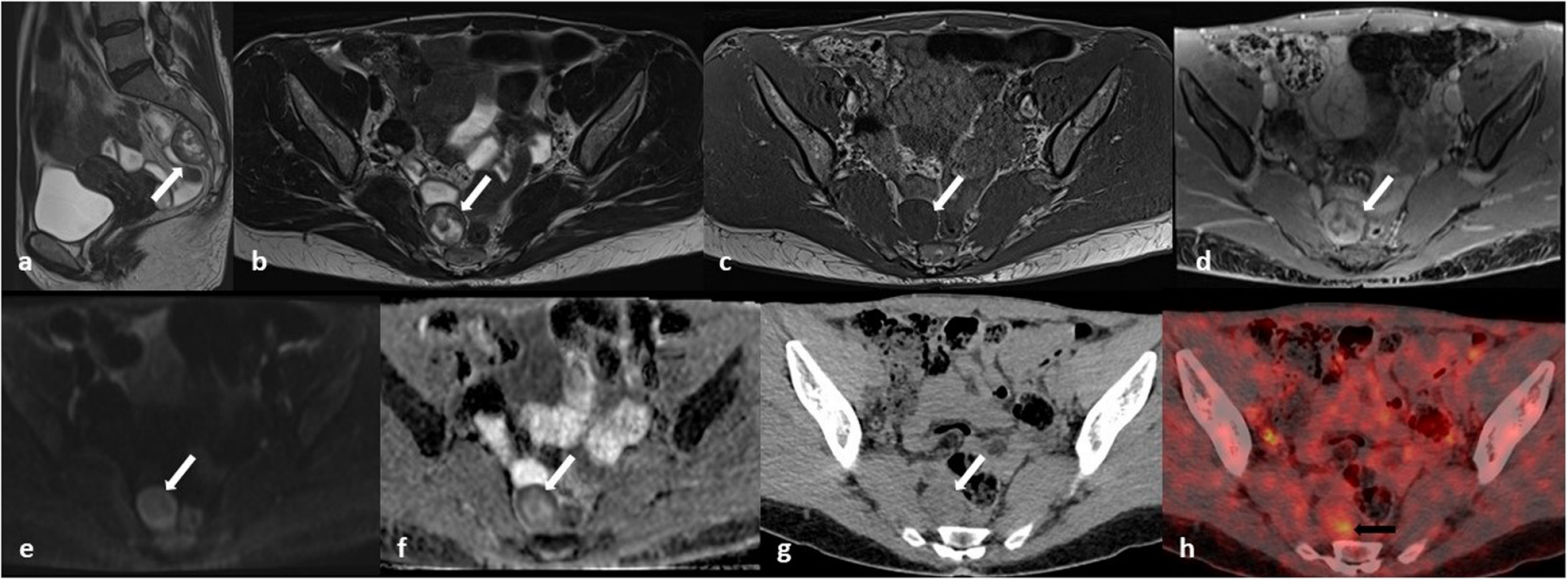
Retroperitoneal Schwannoma in a 52-year-old woman. a–f MR images show a round encapsulated mass (white arrows) with a central area of hypointense signal and peripheral hyperintensity on T2-weighted images (target sign) (a–b), intermediate signal on T1-weighted images (c), inhomogeneous contrast enhancement on fat-suppressed gadolinium-enhanced T1-weighted images, with a central focal area of contrast enhancement surrounded by peripheral hypointensity and an outer area of enhancement (d); moderate signal on diffusion-weighted images with the highest *b* value (e) associated with a peripheral hypointensity on apparent diffusion coefficient (ADC) map (f). g On non-enhanced CT, the lesion appears homogeneously isodense to the muscles. h On 18F-FDG PET/CT, it shows a focal area of hypercaptation (black arrow)
